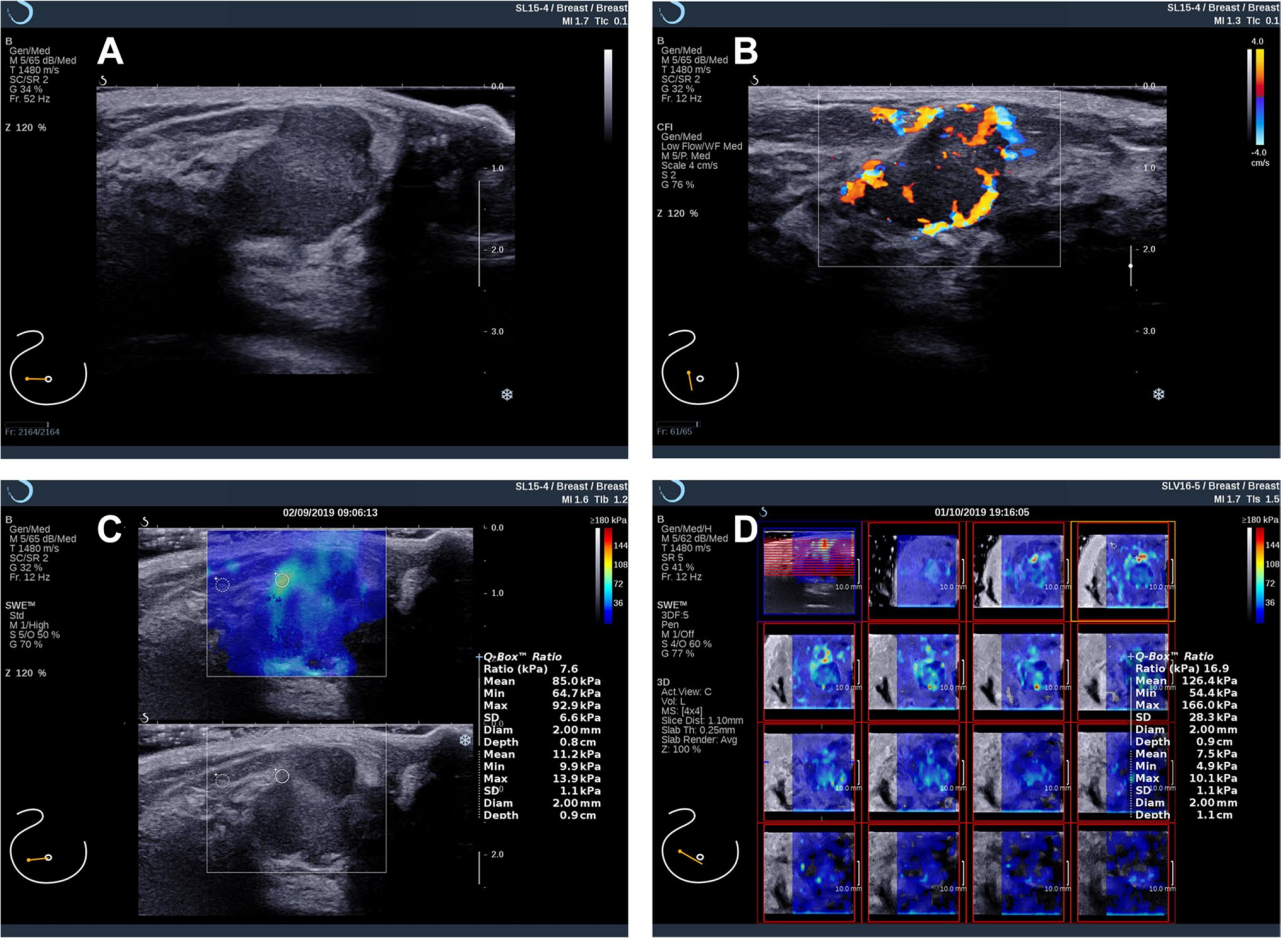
A 46-year-old female participant with ductal carcinomas in situ (DCIS) in the right breast. Standard breast US assessed to BI-RADS 4a using B-mode ultrasound (A) and color Doppler (B) examination. C A mean elasticity of 85.0 kPa according to 2D SWE only indicated downstaging of the lesion to BI-RADS 3. D A mean elasticity of 126.4 kPa in 2D + 3D SWE assessment indicated that biopsy should be performed in this lesion
